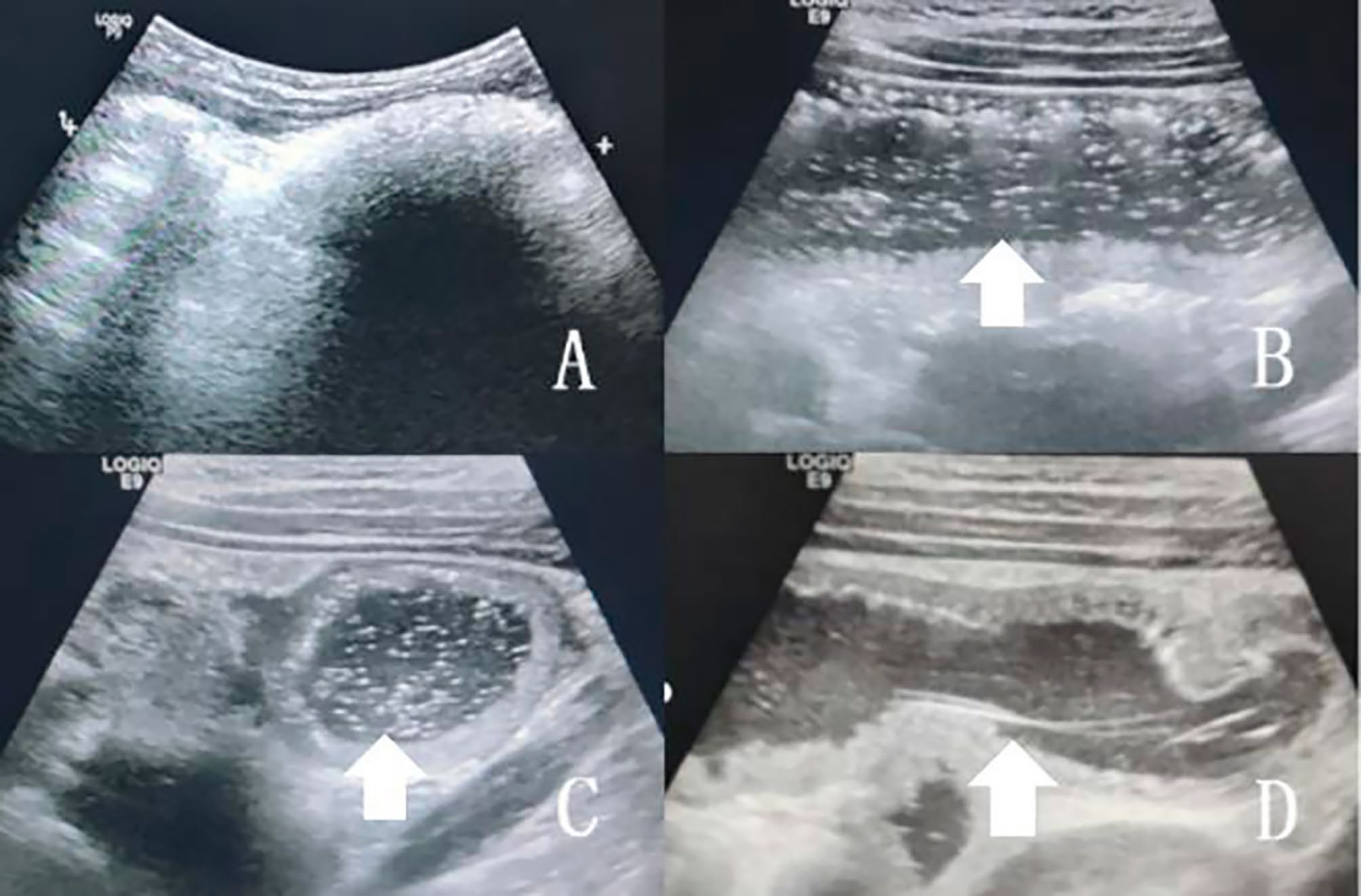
(A) Ultrasonography showing a hyperechoic arc-like surface of the gastric mass. (B, C) Multiple punctate hyperechoic points and intestinal wall thickening on the longitudinal axis and cross section of the bowel. (D) Fine filamentous hyperechoic structure in the longitudinal intestinal cavity
